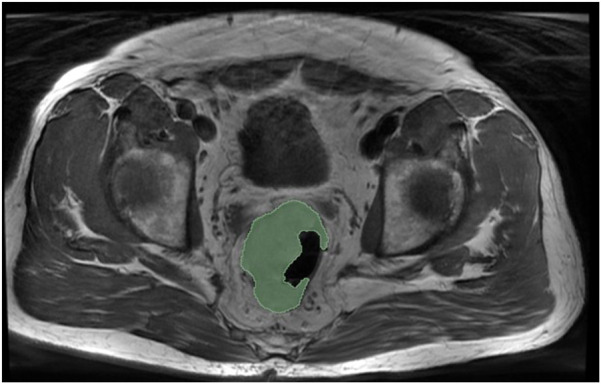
Axial T1-weighted MRI sequence of the pelvic region in a colorectal cancer patient. The green area indicates the segmented tumor region of interest (ROI), from which radiomics parameters were computed. This segmentation method was then applied across all MRI sequences used in the study.
